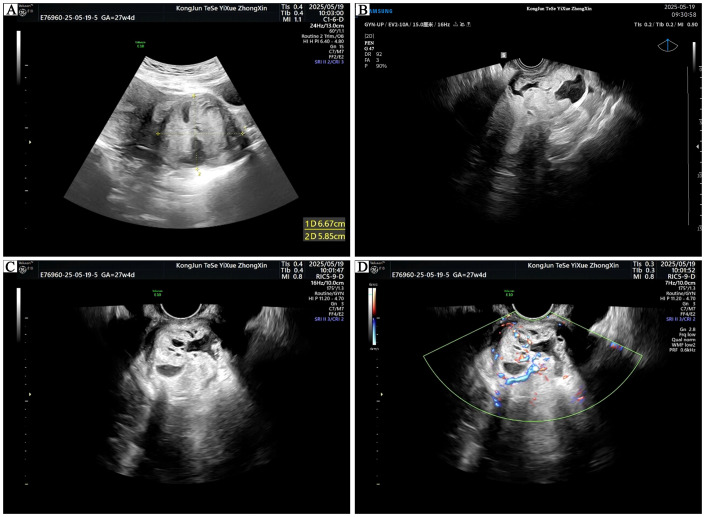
Transvaginal ultrasound findings in a patient with PJS and G-EAC. (A) Transverse review reveals a complex cystic-solid cervical lesion measuring 6.9×5.9 cm. (B) The lesion demonstrates indistinct boundaries with the lower anterior uterine wall. (C) Interventional ultrasound characterizes the lesion as having variably sized cystic areas, irregular margins, and disruption of the cervical serosa. (D) Color Doppler imaging demonstrates marked hypervascularity within the solid components of the lesion.
